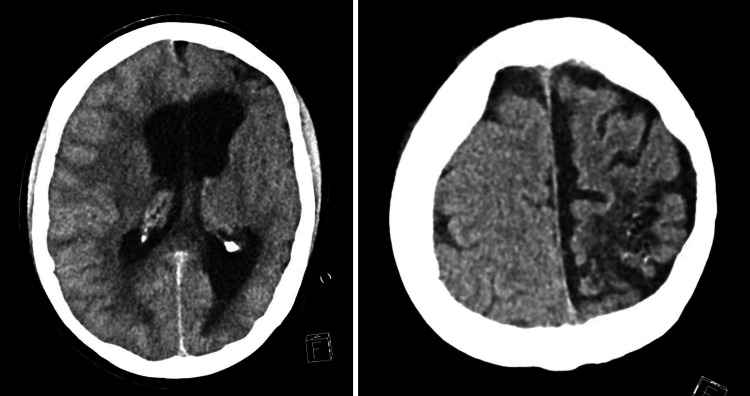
Initial CT head without contrast showing mass effect in the right cerebral hemisphere causing right to left midline shift and cerebral edema
